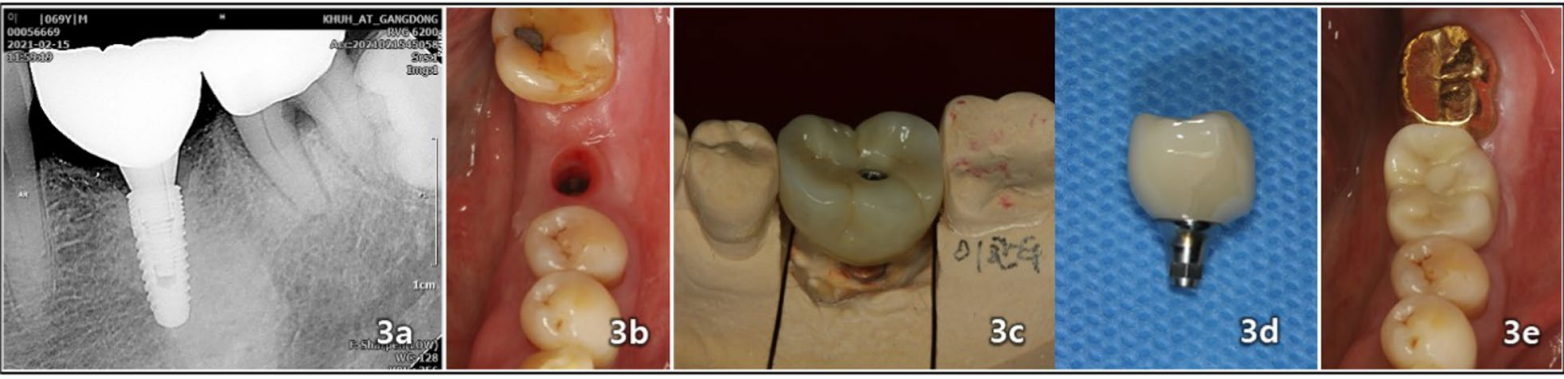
Insertion of the final restoration and clinical follow-up included the following: a A nine-year follow-up involved a periapical radiographic image of the operation site after the placement of the Monolithic Zirconia crown as the definitive restoration. b This was conducted prior to the insertion of the definitive implant restoration on February 15, 2014. c, d The definitive restoration was accomplished with an implant-fixed, screw-fastened Monolithic Zirconia crown. e One year after the insertion of the definitive implant restoration, a follow-up was conducted
